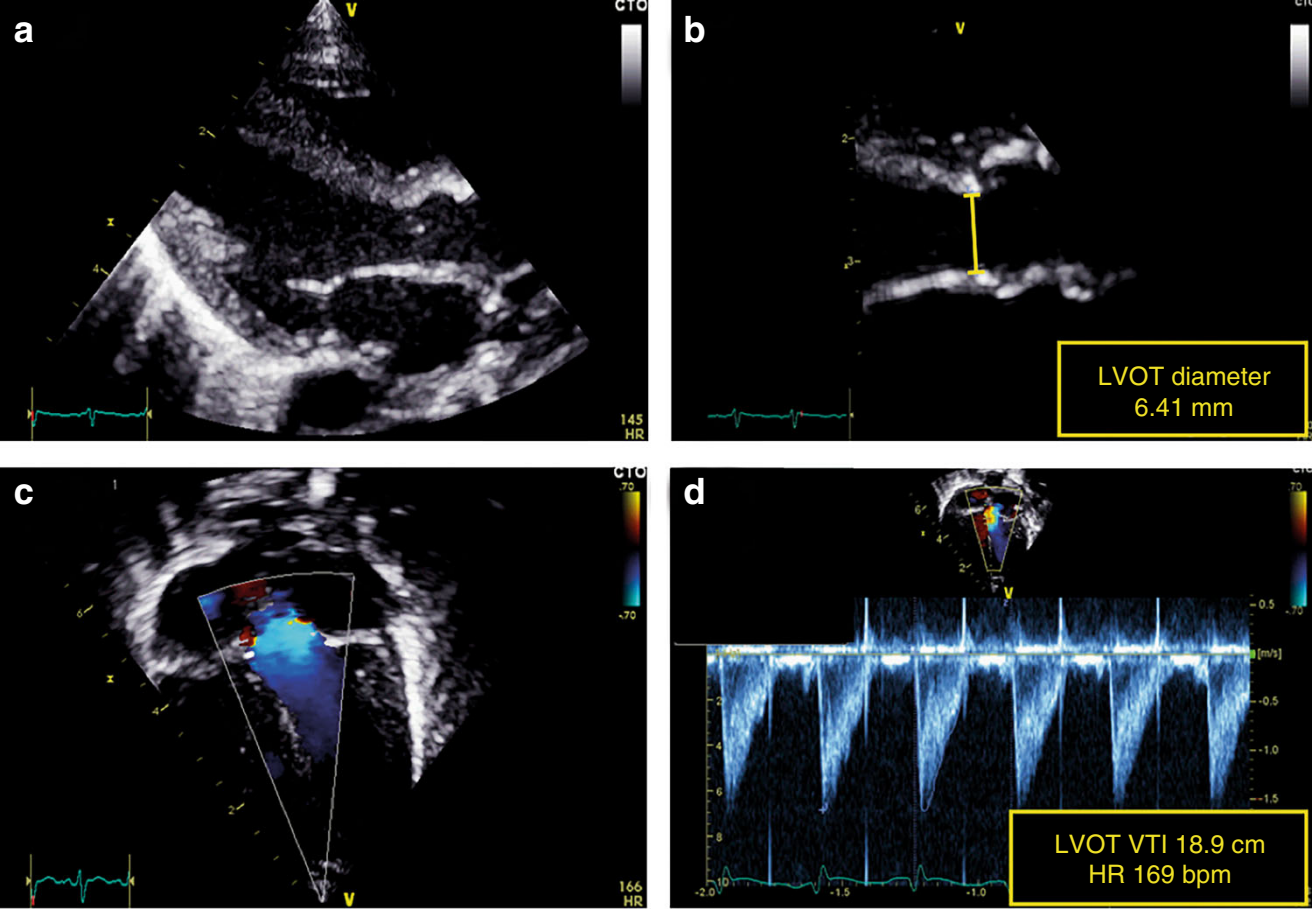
Assessment of left ventricular output (LVO). LV outflow tract diameter is measured from the parasternal long-axis view (a). The diameter measurement should be performed at the hinge points of the aortic valve at end-systole (b). The velocity time integral (VTI) in the LV outflow tract should be assessed in the apical five-chamber view or apical long-axis view using pulsed-wave Doppler with the sample volume just below the aortic valve (c). The pulsed wave Doppler recording is paused to obtain a smooth VTI envelope for exact tracing of the signal (d)
